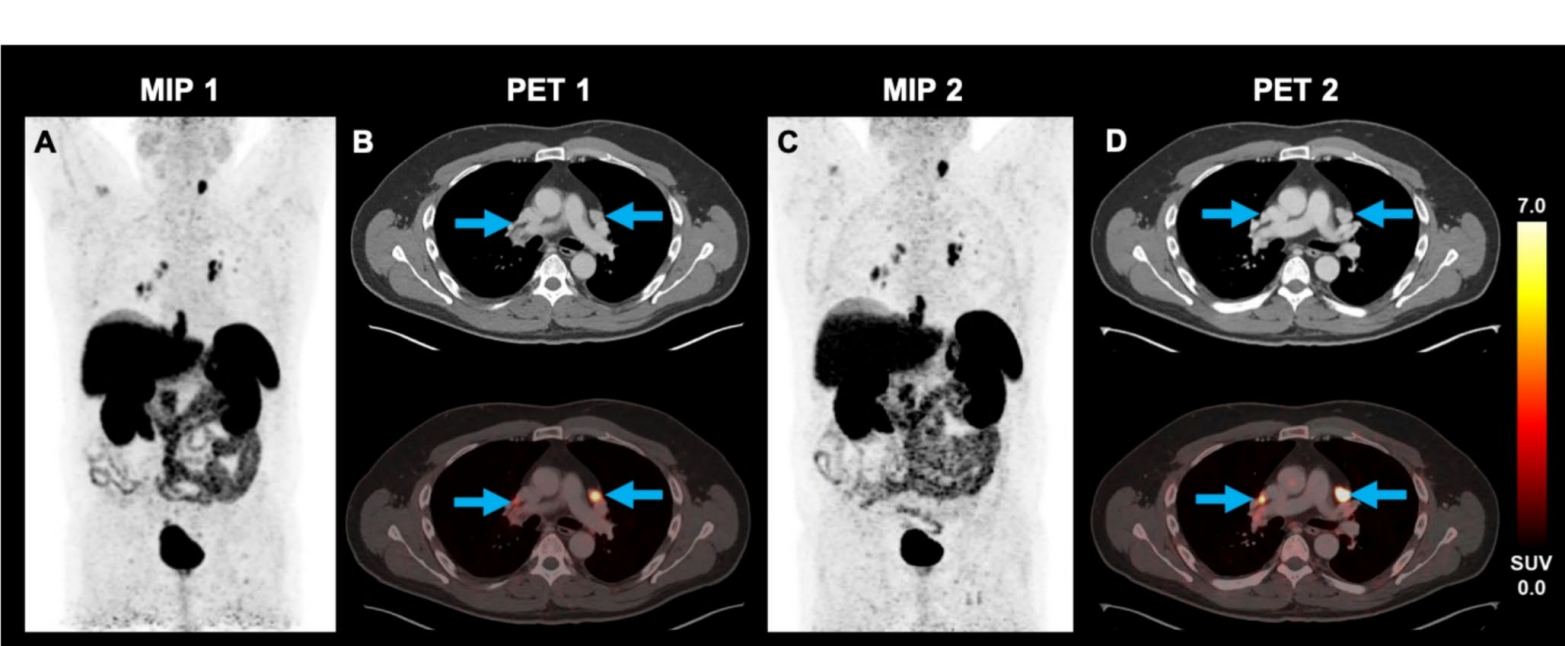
A 52-year-old male patient diagnosed with medullary thyroid carcinoma underwent thyroidectomy and was referred to our department due to a calcitonin level of 10,797 pg/mL. The maximum intensity projection (MIP) and selected transversal levels of hilar metastases (blue arrows) of the baseline and follow-up PET are shown. The baseline [^18^F]SiTATE PET/CT showed a TTV of 21.5 mL (A-B). Metastases were located cervical, mediastinal, bipulmonary and hilar. After 11 months of a watchful waiting strategy both the calcitonin level (12,329 pg/mL) and the TTV (33.7 mL) on the follow-up [^18^F]SiTATE PET/CT increased (C-D)
